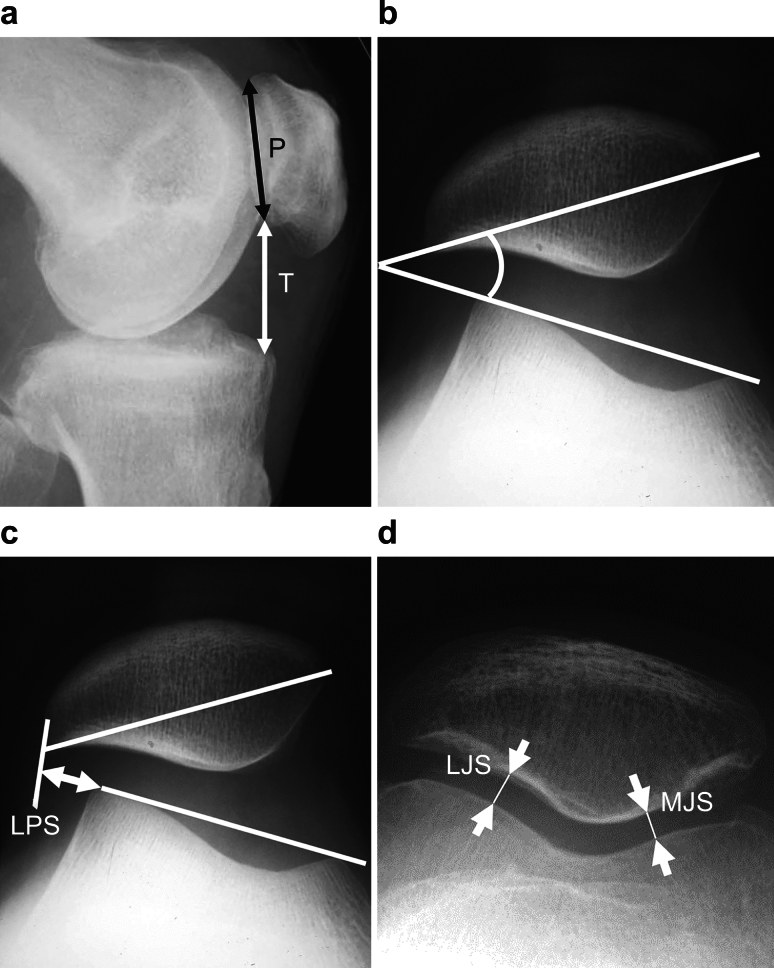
Measurement methods of the patellofemoral joint. a Caton–Deschamps index (CDI) = *T*/*P*, where *P* is the patellar articular surface length and *T* is the distance from the anterior angle of the tibial plateau to the most inferior aspect of the patella articular surface. b Tilting angle (TA): the angle between the line intersecting the widest bony structure of the patella and the line tangentially passing the anterior surface of the femorl condyle. c Lateral patellar shift (LPS): the distance between the summit of the lateral femoral condyle and the point where a line from the lateral edge of the patella perpendicular to the line passes through the summit of the femoral condyles crosses that line. d Medial joint space/lateral joint space (MJS/LJS): most narrowed space of the patellofemoral joint. For LPS, MJS, and LJS, the value divided by the width of the patella (p) was also measured to correct the X-ray magnification (LPS/p, MJS/p, LJS/p)
